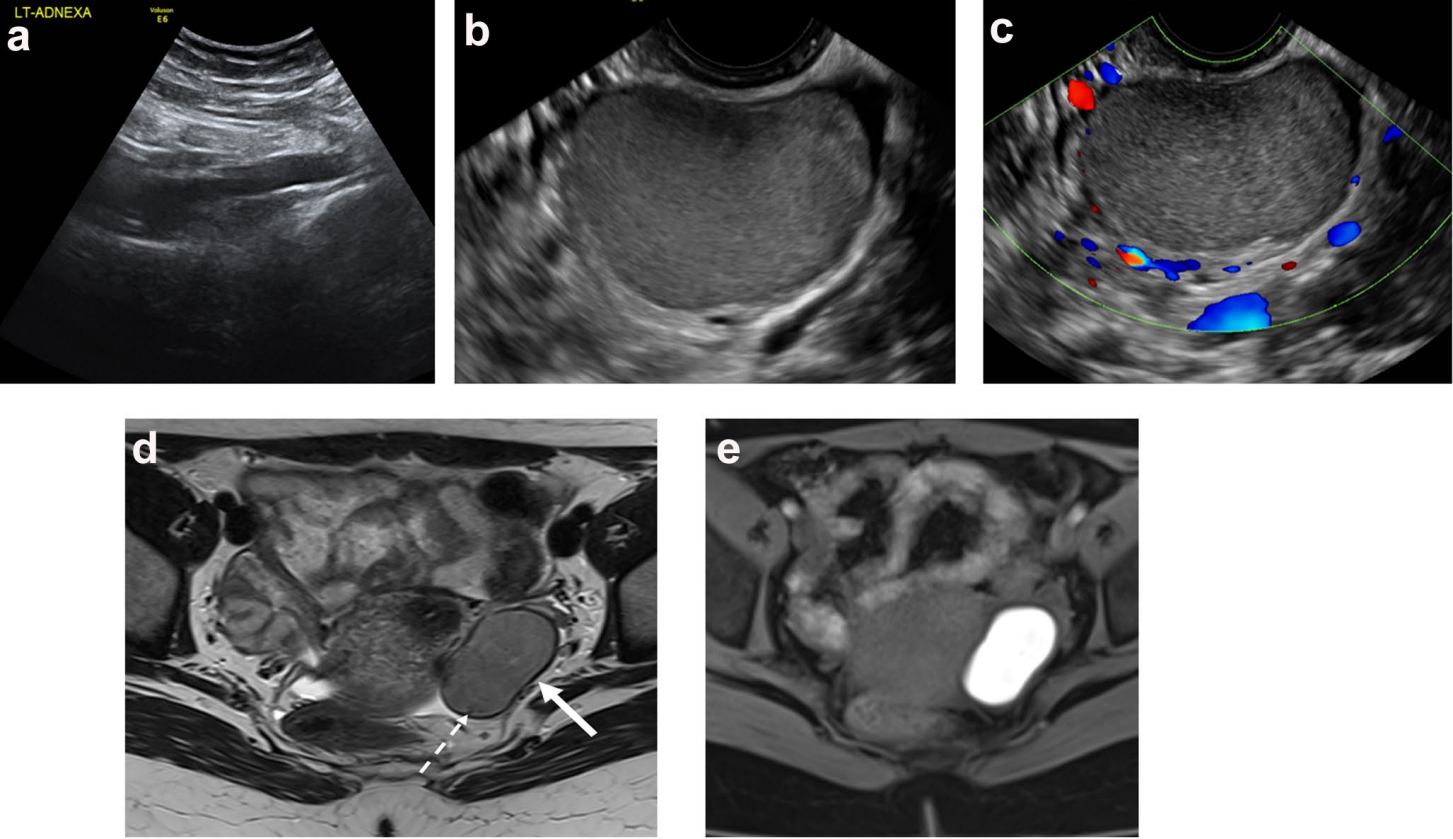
18 year-old woman with left-sided pelvic pain. a The ovaries were not identified by transabdominal ultrasound technique. b, c The patient was amenable to transvaginal ultrasound which showed a unilocular left ovarian cystic lesion with homogeneous low-level internal echoes and no internal vascular flow with color Doppler. d The lesion shows characteristic T2 shading on T2W MR image (solid white arrow) with a peripheral dark spot (dashed white arrow). e The lesion shows characteristic homogeneous hyperintense signal on T1W image with fat suppression. Patient underwent laparoscopy 2 months later with cystectomy of the endometrioma. Superficial endometriosis was noted in the posterior cul-de-sac
